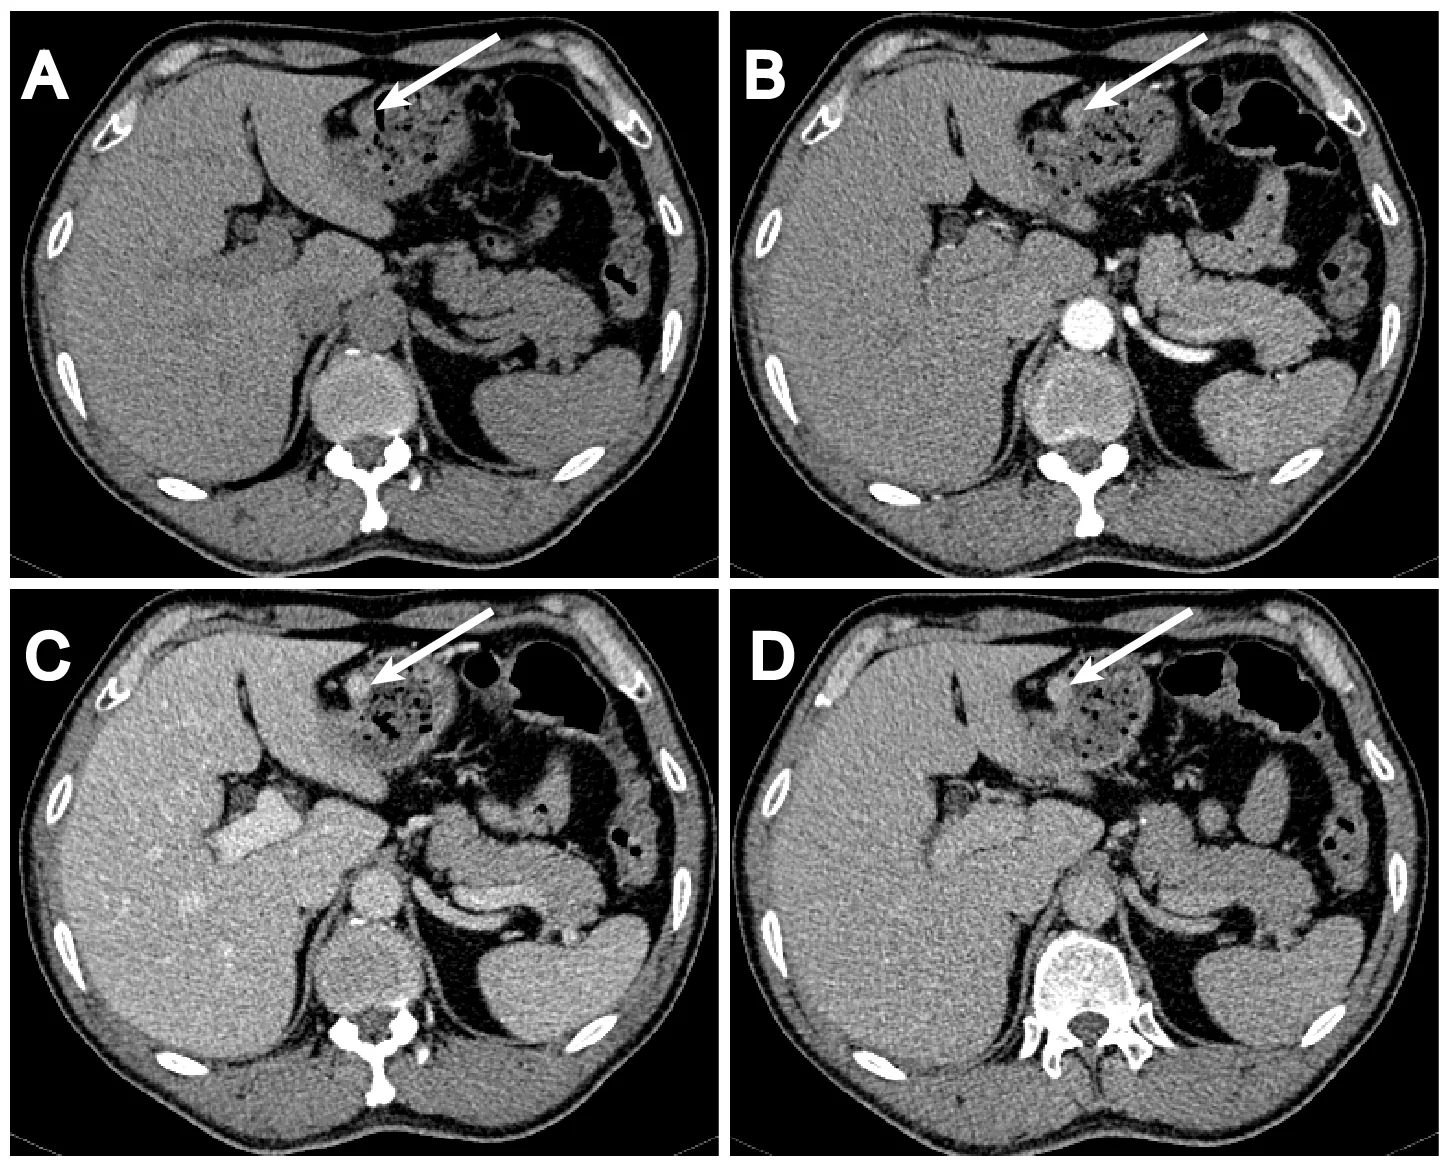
Unenhanced and contrast-enhanced CT findings of the lesion. (A) Unenhanced CT shows a well-circumscribed nodule (11 × 9 mm) in the anterior wall of the gastric antrum. (B–D) Contrast-enhanced CT demonstrates marked enhancement in the arterial phase with persistent enhancement in the portal venous and delayed phases. The lesion is indicated by the white arrow.
